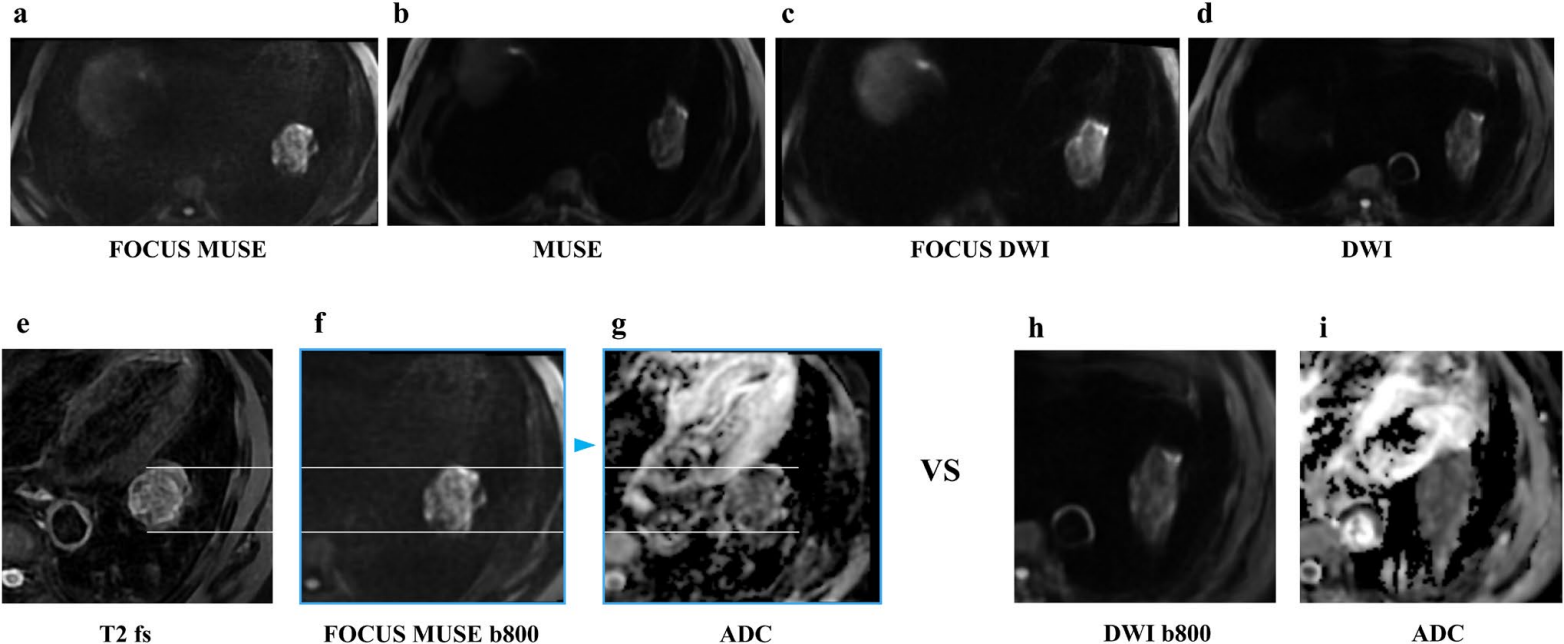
Male, 75 years old, soft tissue mass in the lower lobe of the left lung, approximately 41 mm in size. Pathology confirmed a malignant epithelial tumor with some neuroendocrine differentiation. (a) FOCUS MUSE DWI (*b* = 800 s/mm^2^); (b) MUSE DWI (b = 800 s/mm2); (c) FOCUS DWI (b = 800 s/mm^2^); (d) SS DWI (b = 800 s/mm2); (e) magnified lesion image on T2WI; (f) magnified lesion image on FOCUS MUSE b800; (g) magnified lesion image on FOCUS MUSE ADC; (h) magnified lesion image on DWI b800; and (i) magnified lesion image on DWI ADC.
